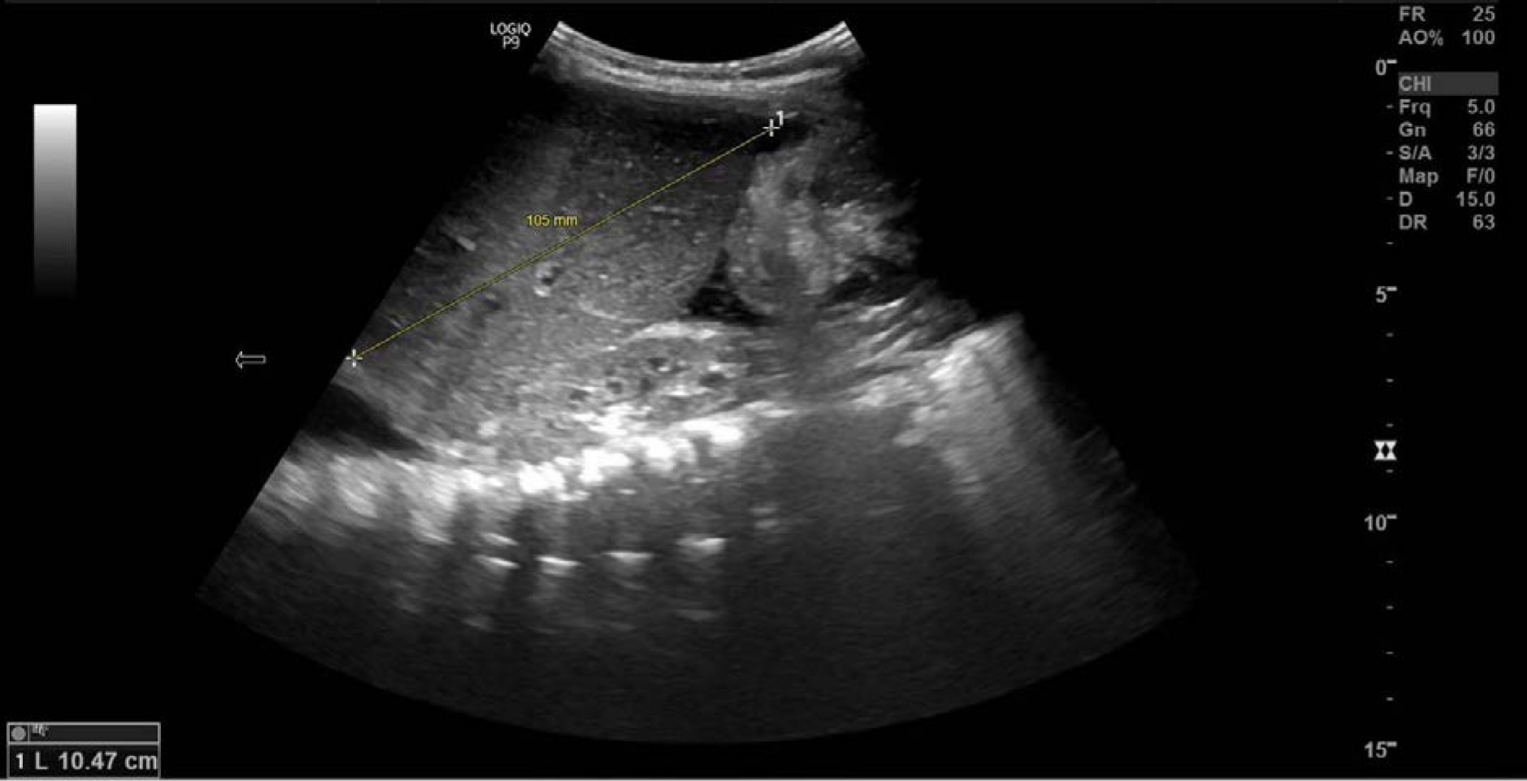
Abdominal ultrasound showing a 10.5 cm liver span along the midclavicular line. The liver has a homogenous texture and a smooth regular outline.
